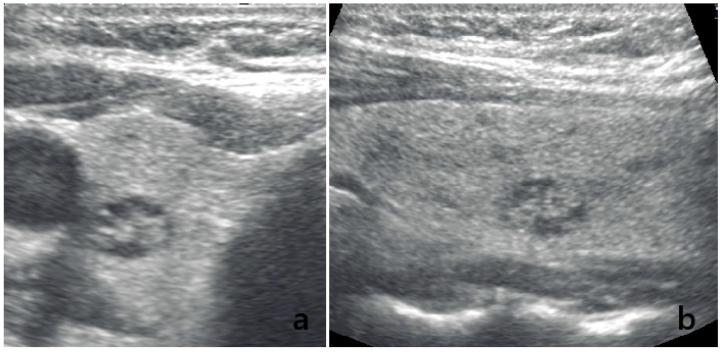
Ultrasound images of a benign thyroid nodule with discrepant ACR TI-RADS classifications between the radiologist and GPT-5.0. (a,b) show two orthogonal ultrasound views of the same nodule. The radiologist classified the lesion as TI-RADS 2, noting its isoechoic appearance, smooth margins, and absence of suspicious sonographic features, all of which are consistent with a benign profile. In contrast, the GPT-5.0 model assigned the nodule a TI-RADS 4 score.
